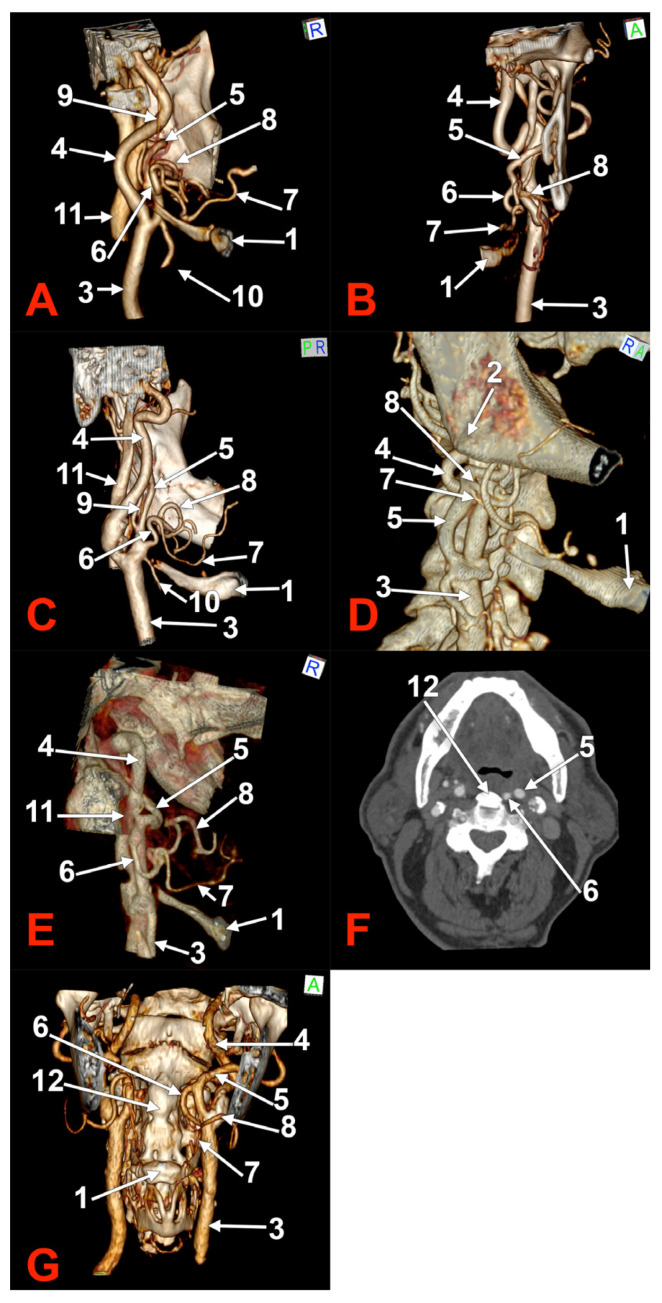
Morphological variants of unilateral left linguofacial trunks. Computed tomography angiograms. Three-dimensional volume renderings. Anterior (B,C,G), right lateral (D), and medial (A,C,E) views. Axial slice (F). 1. hyoid body; 2. gonial angle; 3. common carotid artery; 4. internal carotid artery; 5. external carotid artery; 6. linguofacial trunk; 7. lingual artery; 8. facial artery; 9. ascending pharyngeal artery; 10. superior thyroid artery. 11. internal jugular vein; and 12. ossified anterior longitudinal ligament.
